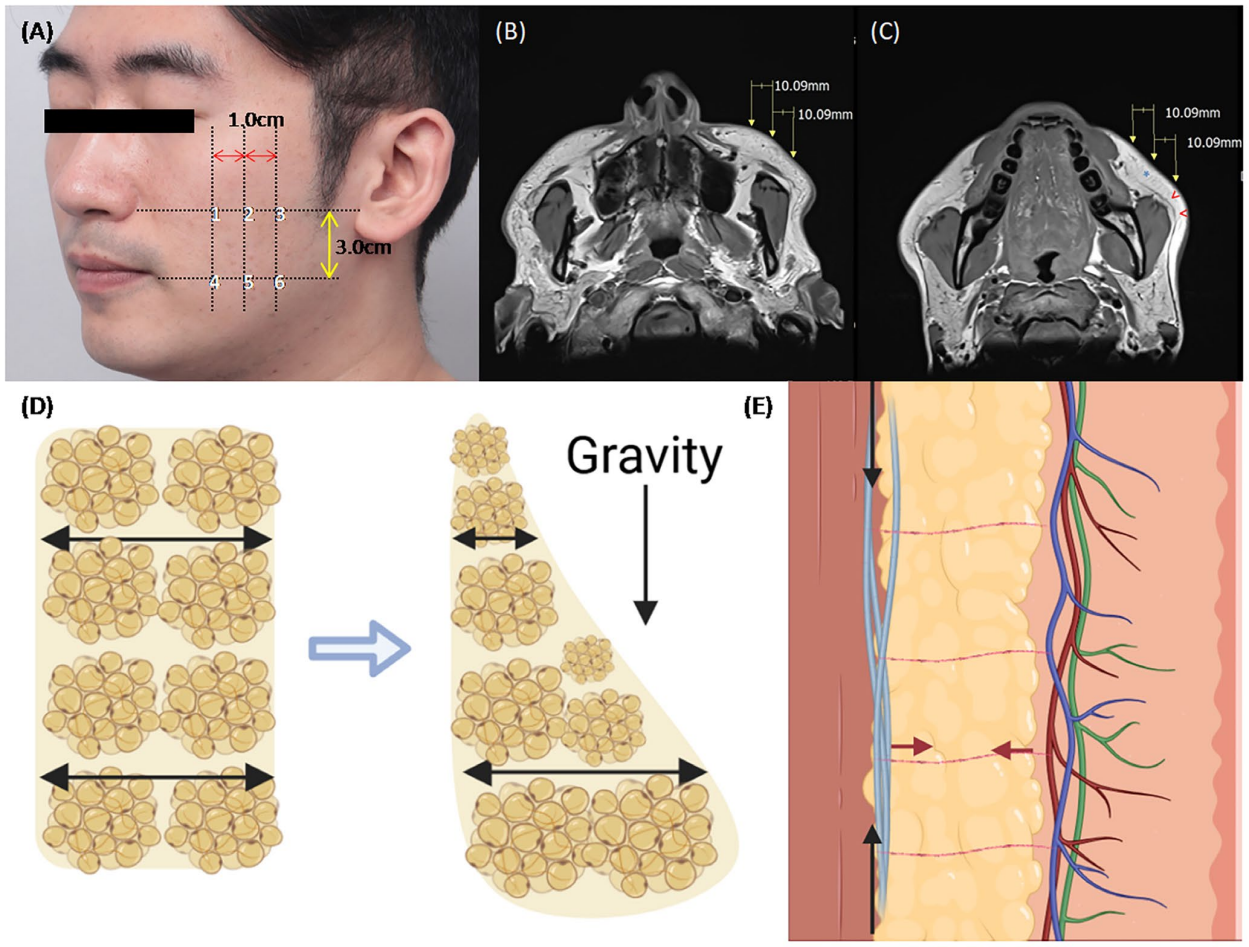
(A–C) 6 Points of measurements, red arrow head showed superficial musculoaponeurotic system and blue asterisk showed superficial fat layer with vertical fibrous septae on T1‐weighted MRI. (D) Superficial fat gliding to inferior forming droplet‐like shape in the sagittal plane. The ratio of fat thickness (upper over lower fat) become smaller with aging. (E) Superficial musculoaponeurotic system (SMAS) (blue line) contracts antigravital direction (black arrow) while fibrous septae (red line) contracts vertical to SMAS (red arrow).
